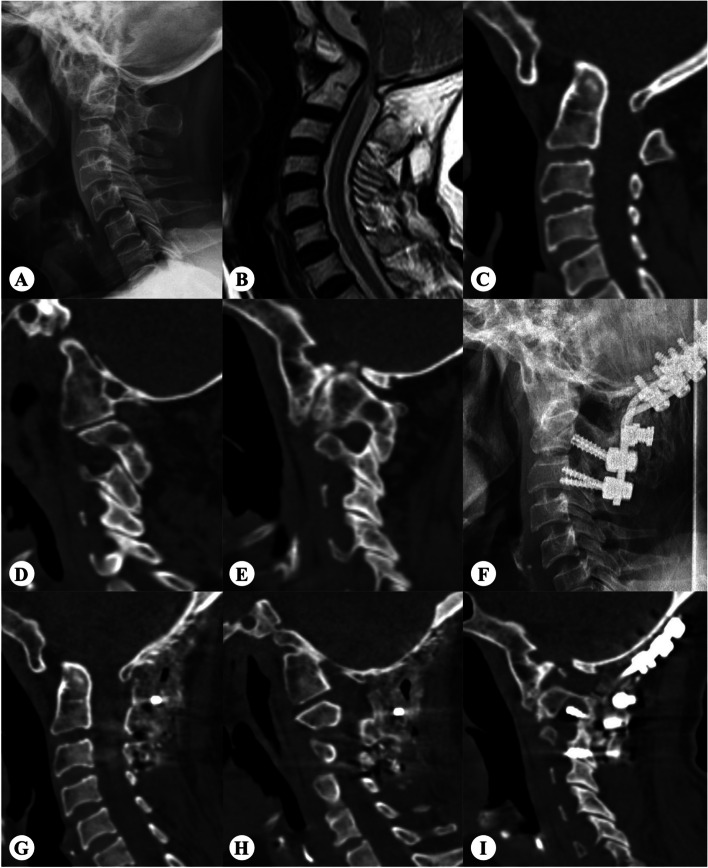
A 65-year-old female complained of numbness of limbs, unsteady walking, and neck pain. A-C Preoperative X-ray, MRI and CT three-dimensional reconstruction showed BI with AAD. D Preoperative CT showed right SSA was − 19.95˚ and (E) left SSA was − 66.52 ˚. Thus, the minimum-SSA was − 66.52 ˚. F-G Postoperative X-ray and CT three-dimensional reconstruction showed horizontal RD was not satisfactory. AAD was 6.25 mm, and RD was 51%. H The right LAA did not obstruct the horizontal reduction. I However, the left SSA was too small, which obstructed the horizontal reduction
